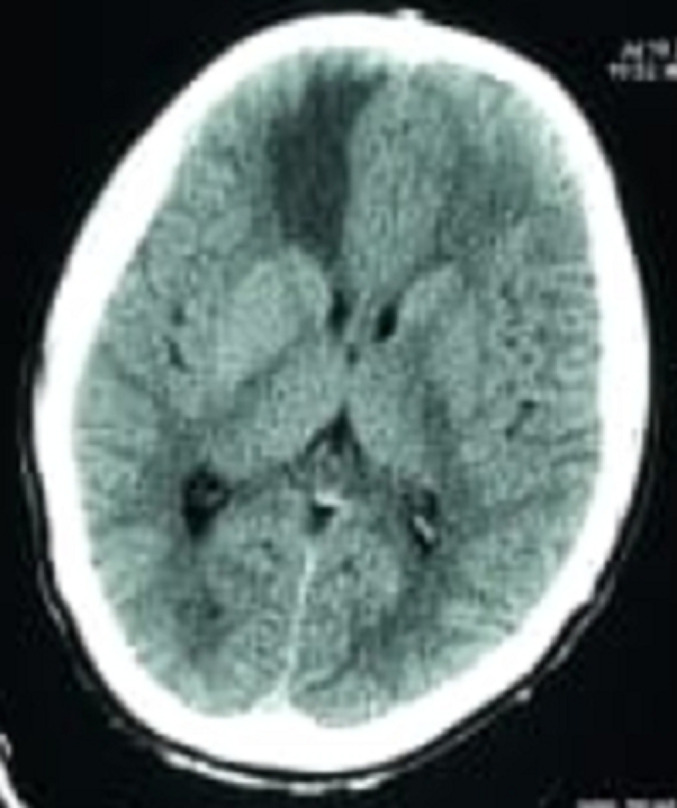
CT scan of a slightly rounded hyper density process of the extra-axial front anterior skull base sitting at the olfactory groove area with significant hypo density peri-lesional oedema on the toe finger more marked on the right and hyperostosis in regard of the lesion
